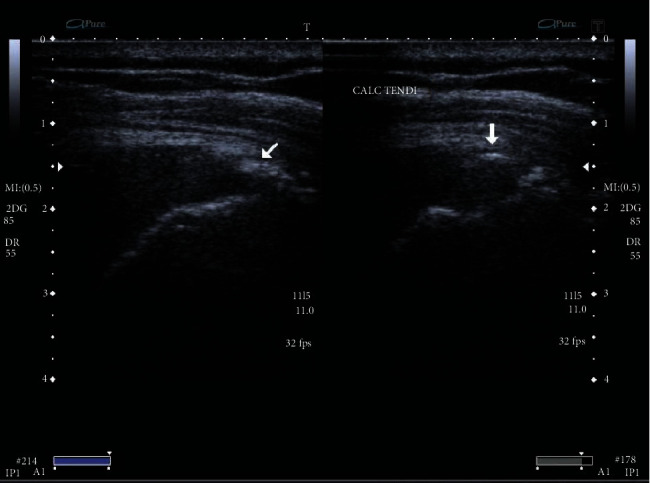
Ultrasonographic changes in calcified supraspinatus tendon (ESWT-RPT group).
